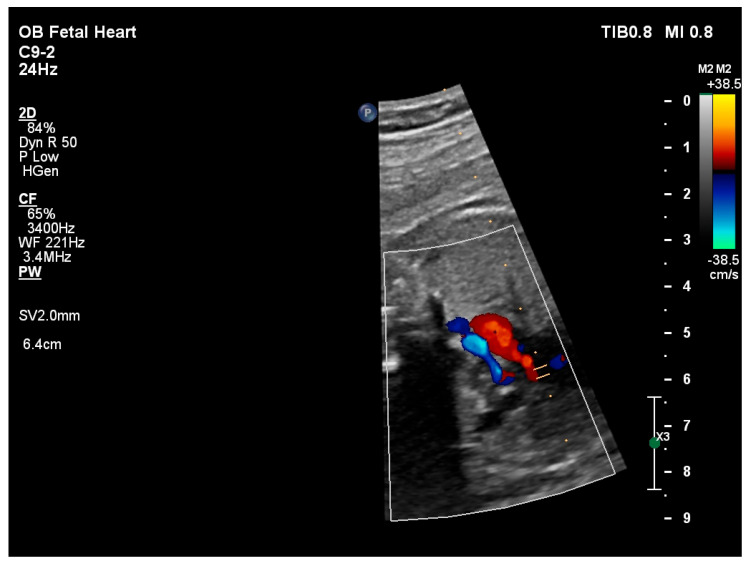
Three-vessel view on fetal echocardiography at 32 weeks’ gestation demonstrating retrograde filling of the ascending aorta.
